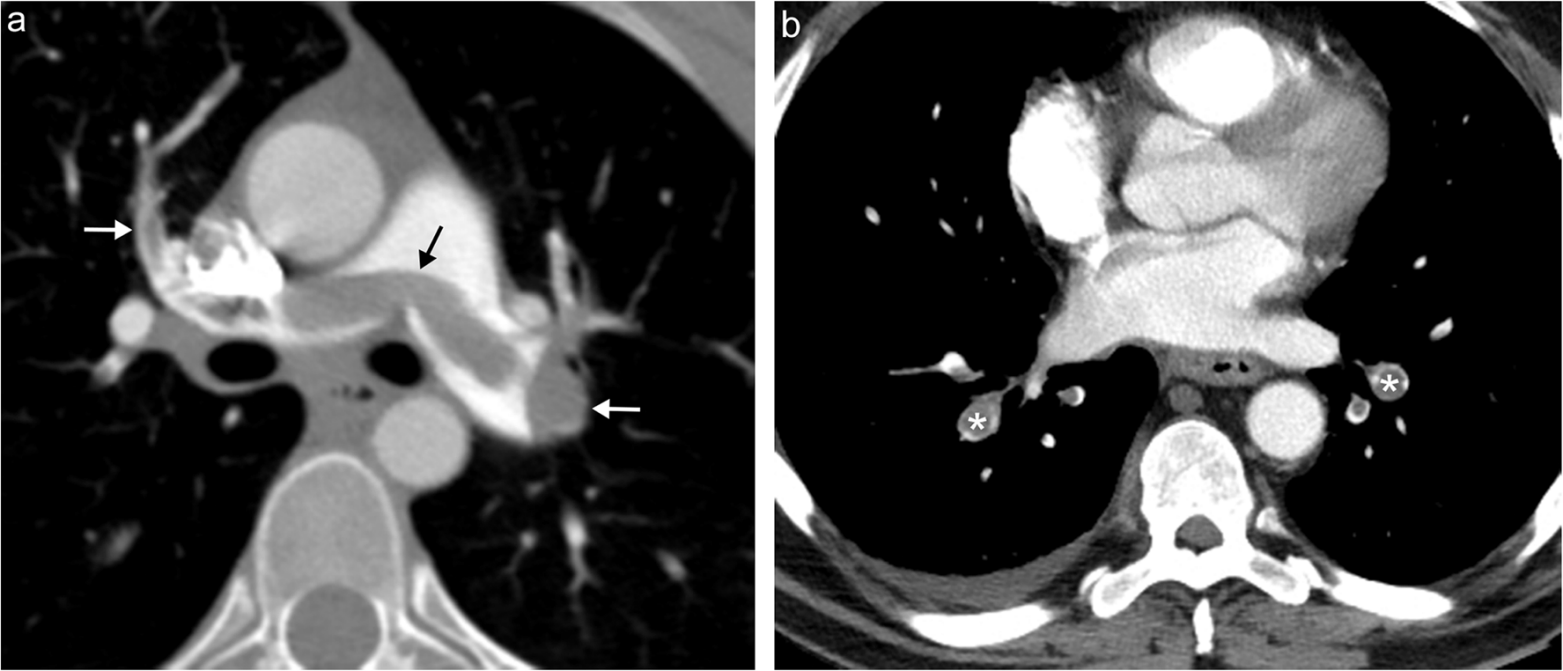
Two patients with acute pulmonary emboli. a Axial contrast-enhanced CT image shows a large saddle embolus of the main pulmonary artery (*black arrow*) as well as lobar/segmental involvement of the right and left pulmonary arteries (*white arrows*). b Axial contrast-enhanced CT image of another patient with bilateral acute pulmonary emboli demonstrate the central focal filling defect within bilateral segmental pulmonary arteries which is rimmed by contrast (*)
